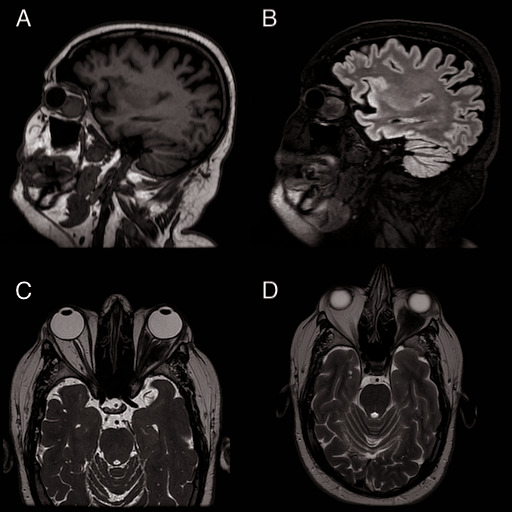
Magnetic resonance imaging (MRI) of the orbit and brain without contrast agent revealed an intraconal mass, whose maximum dimensions are 24.7/ 25.3/ 27.3 mm (in craniocaudal, anteroposterior, and transverse planes), that surrounds the optic nerve on its entire intra-orbital path, with obliteration of adjacent fat planes in the left orbit. A. High resolution three-dimensional T1-weighted gradient image (BRAVO), sagittal plane. B. T2-weighed volume sequence (CUBE) with fluid-attenuated inversion recovery (FLAIR) contrast, sagittal plane C. T2-weighed volume sequence (CUBE), axial plane D. T2-weighed fast recovery fast spin echo sequence (FRFSE), sagittal plane
